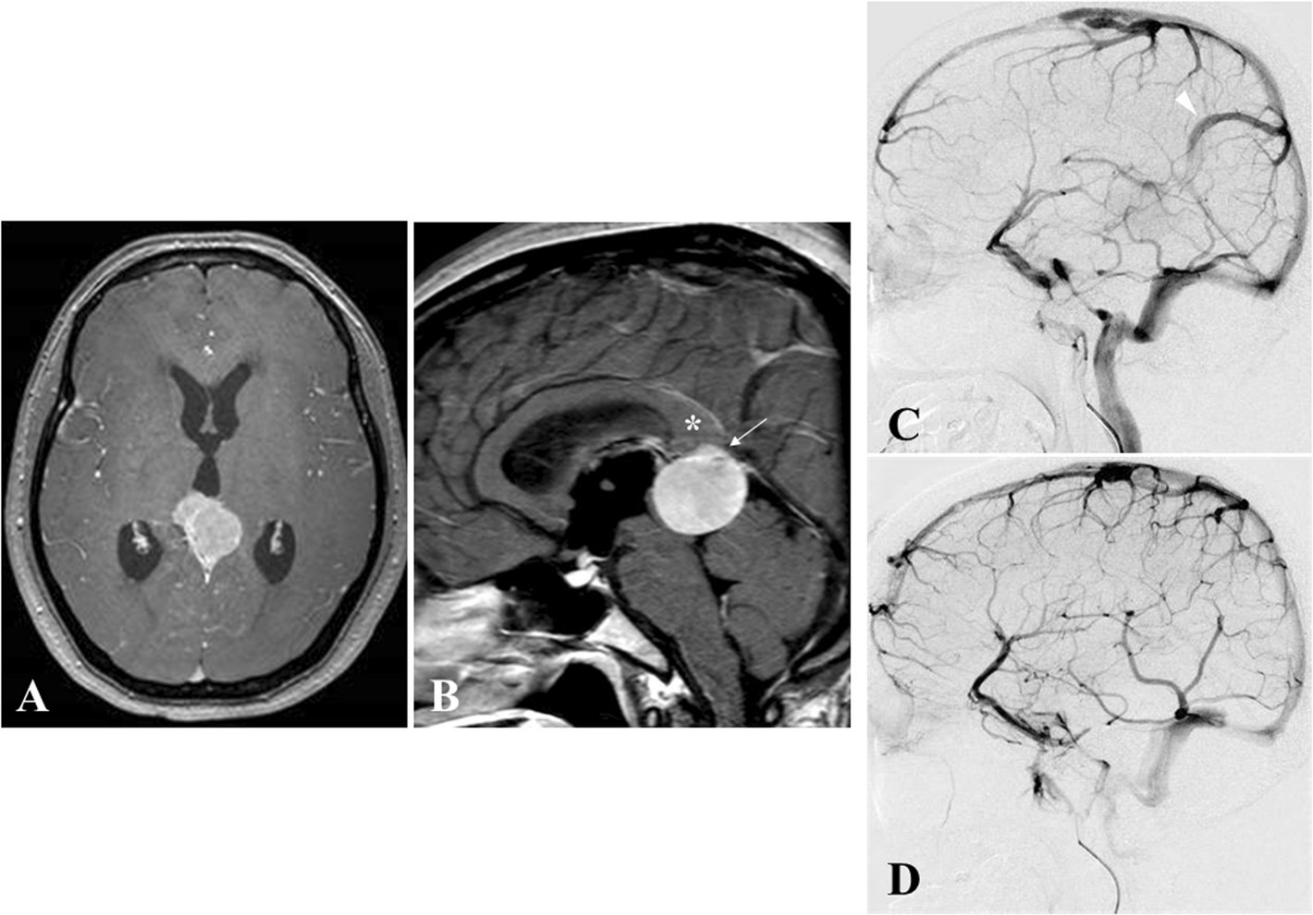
Radiological imaging of the tumor in the pineal region in Case 1. a, b Gadolinium-enhanced T1-weighted magnetic resonance imaging (MRI) in Case 1 shows a well-defined tumor within the quadrigeminal cistern, compressing the dorsal part of the midbrain with resulting mild hydrocephalus (a, axial view, b sagittal view). The vein of Galen (*white arrow*) and splenium of the corpus callosum (*white asterisk*) are displaced upward and the pineal body cannot be identified. c, d Preoperative internal cerebral angiography (ICAG) shows occlusion of the vein of Galen and development of a large collateral venous channel (*white arrowhead*) on the right medial surface of the occipital lobe, draining into the superior sagittal sinus (c, right venous phase, d left venous phase)
